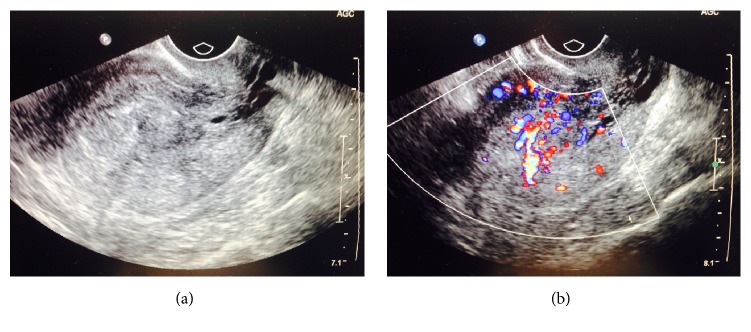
Transvaginal ultrasound examination of pelvic organs. (a) Sagittal view of the uterus with thick mildly heterogeneous endometrium measuring 1.6 cm, without discrete polyp or mass lesion. (b) Possible hypervascular stalk on color Doppler evaluation suggestive of possible endometrial mass.
